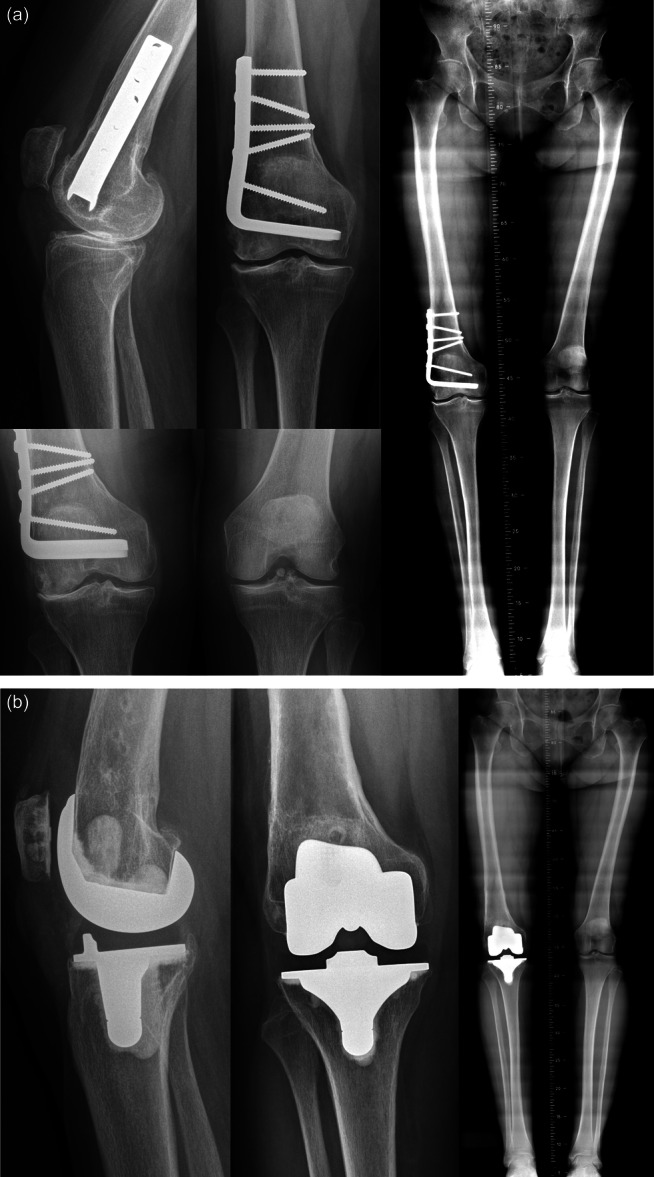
Case report of 56‐year‐old patient operated on lateral opening wedge distal femoral osteotomy (LOW‐DFO), with progression of lateral femorotibial osteoarthritis. (a) Radiographs at 16 years of follow‐up after the DFO showed lateral femorotibial osteoarthritis. (b) Radiographic control after the total knee arthroplasty performed at 16 years post‐DFO.
